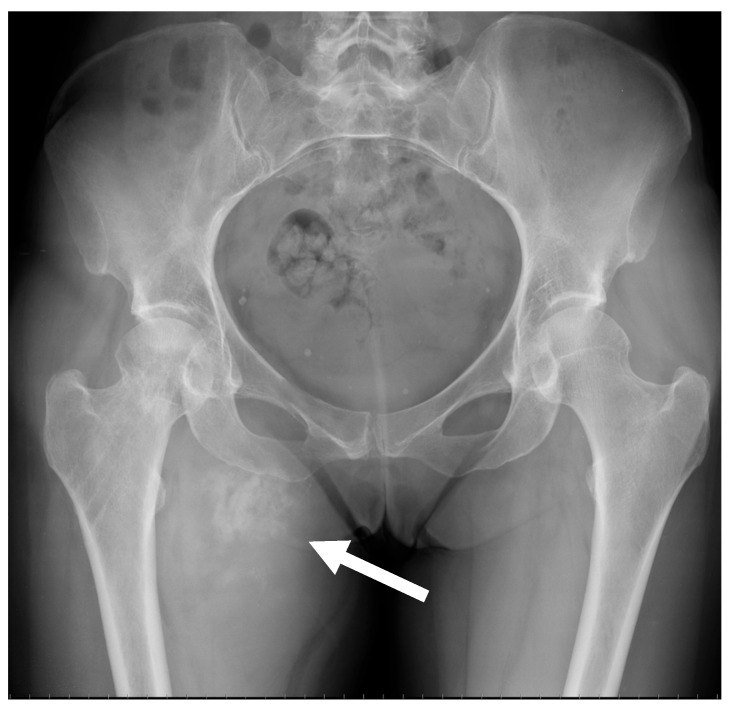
Conventional radiograph of the pelvis of a 51-year-old female diagnosed with extraskeletal osteosarcoma of the right proximal thigh in the abductor region. A mineralized mass with irregular large calcifications/ossifications can be observed (arrow).
